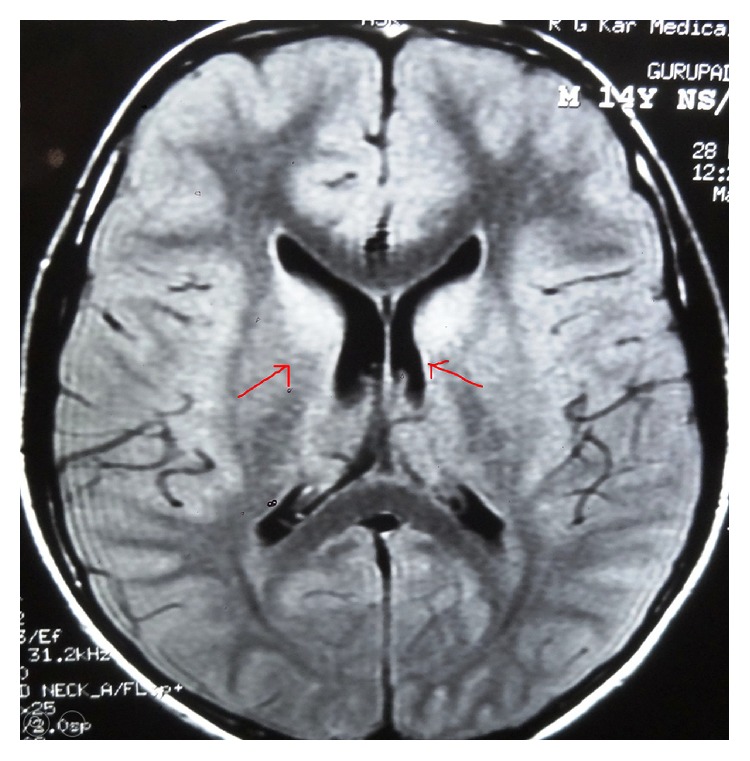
MRI (T2 FLAIR) showing hyperintensities of both caudate nuclei.
